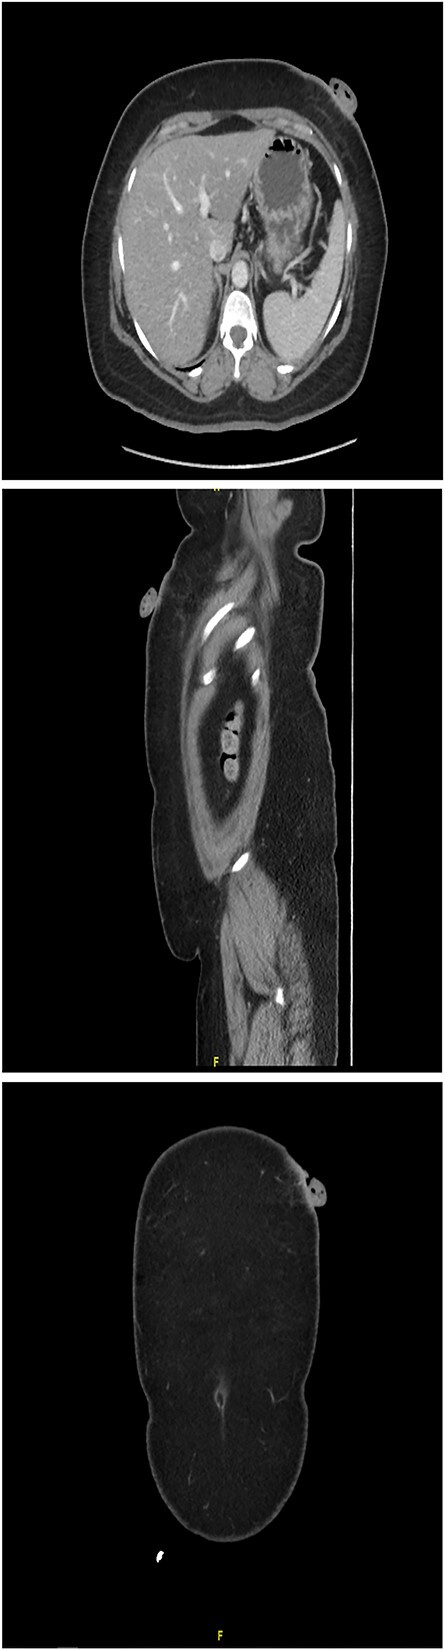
CT scanned abdomen and pelvis showing an anterior left upper abdominal wall skin-based, pedunculated, enhancing soft tissue nodule with a large stalk
